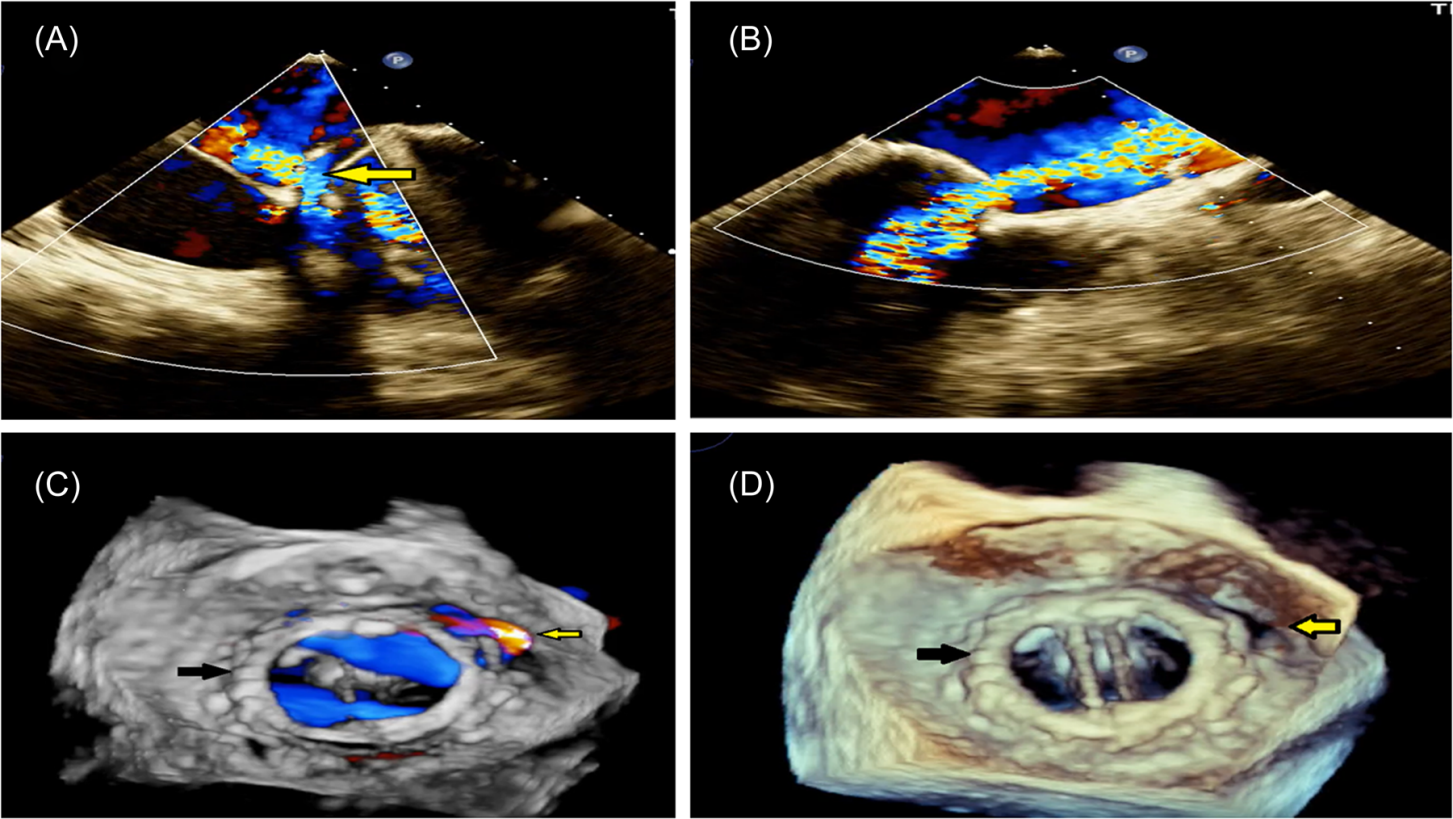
(A) TEE 4 chamber view. Dehiscence of the medial side of MV sewing ring. Severe paravalvular leakage (yellow arrow). (B) TEE parasternal view. Dehiscence of the anterior side of MV sewing ring. Severe paravalvular leakage. (C) 3D TEE color. Surgical view of prosthetic MV (black arrow) with paravalvular leakage (yellow arrow). (D) Surgical view of prosthetic MV (black arrow) with a paravalvular defect in the anteromedial side of MV sewing ring (yellow arrow). TEE, transesophageal echocardiography.
